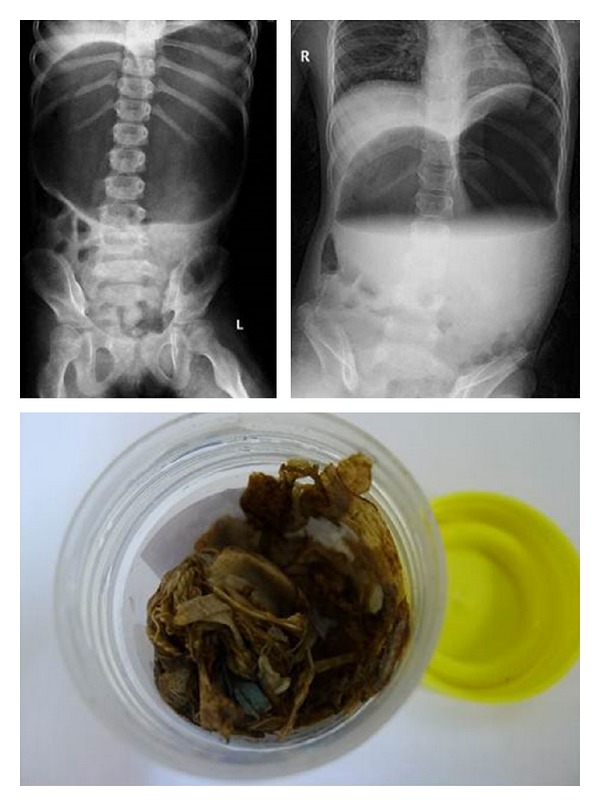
Grossly distended stomach by radiography and debris (gauze and adhesive tape) removed in this child with 46XX del (11).
